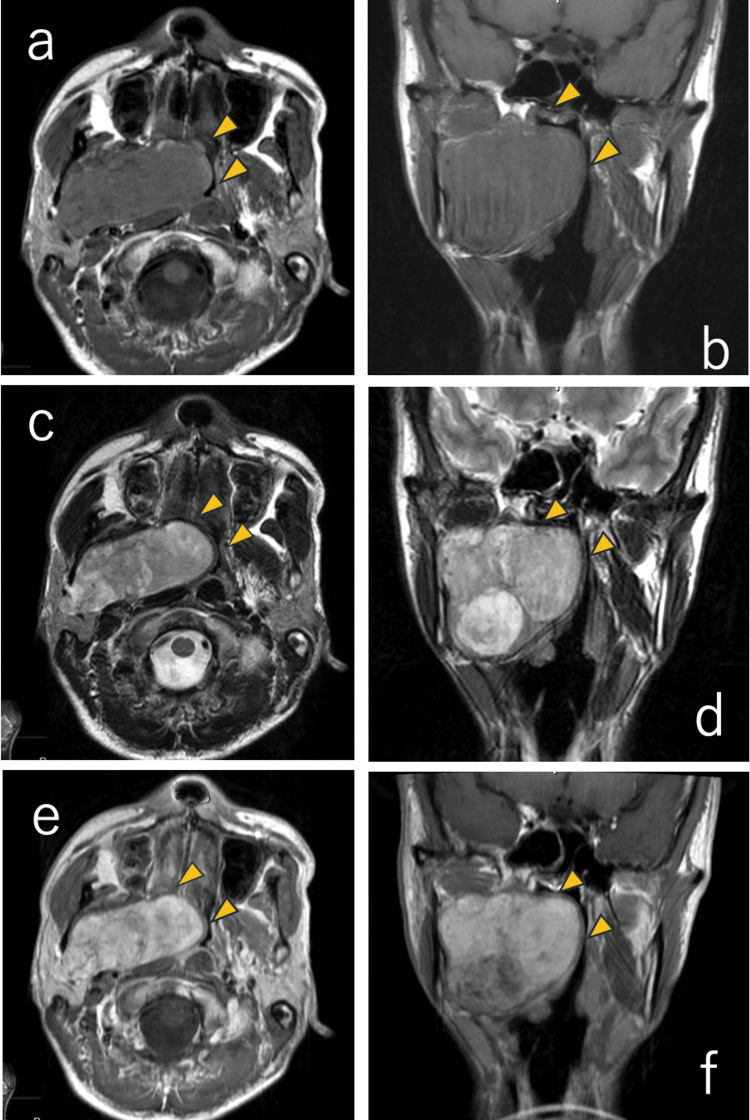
Magnetic resonance imaging reports The MRI indicated an 80-mm-long neoplastic lesion (arrowheads) occupying the oral cavity to the parapharyngeal space. (a) and (b): T1-weighted image; (c) and (d): T2-weighted image; (e) and (f): Gadolinium-enhanced T1 weighted images show mild enhancement
